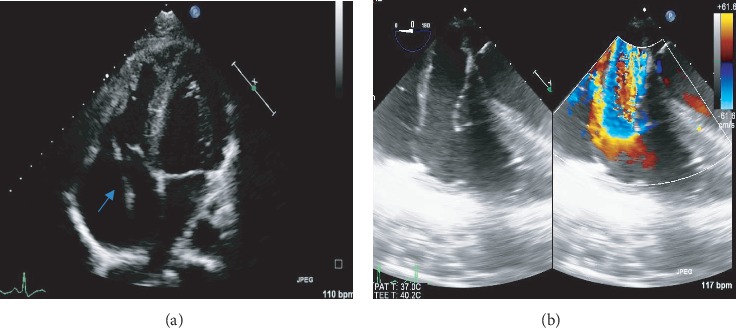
(a) Transthoracic apical 4-chamber echocardiographic view showing flail anterior tricuspid valve leaflet (arrow) and (b) transoesophageal right ventricle long axis views depicting severe tricuspid valve regurgitation.
